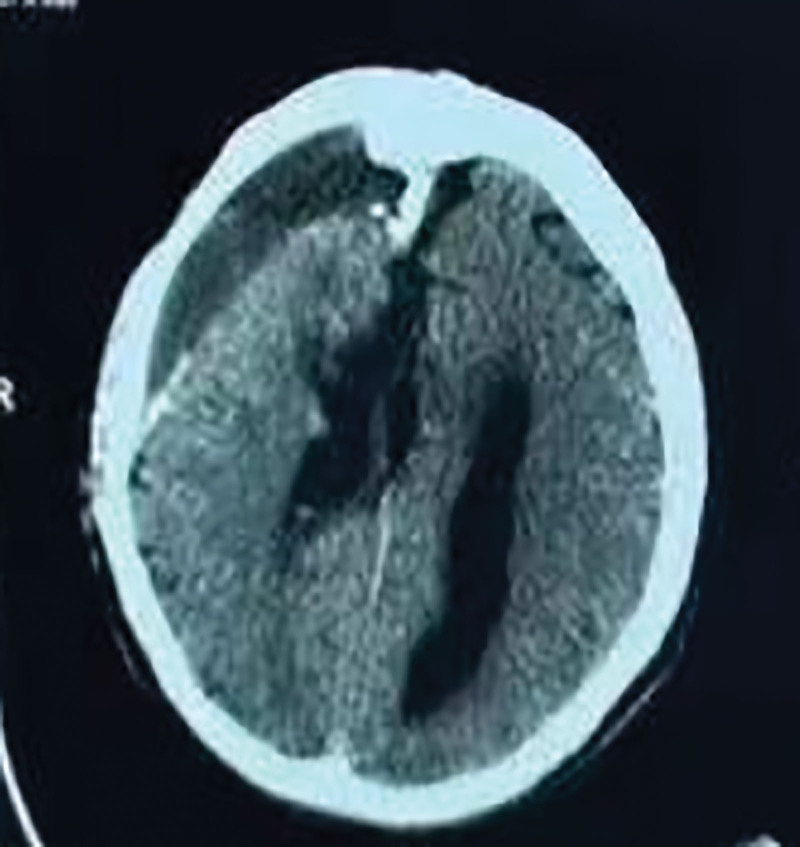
Postoperative follow-up cranial CT. CT = computed tomography.
